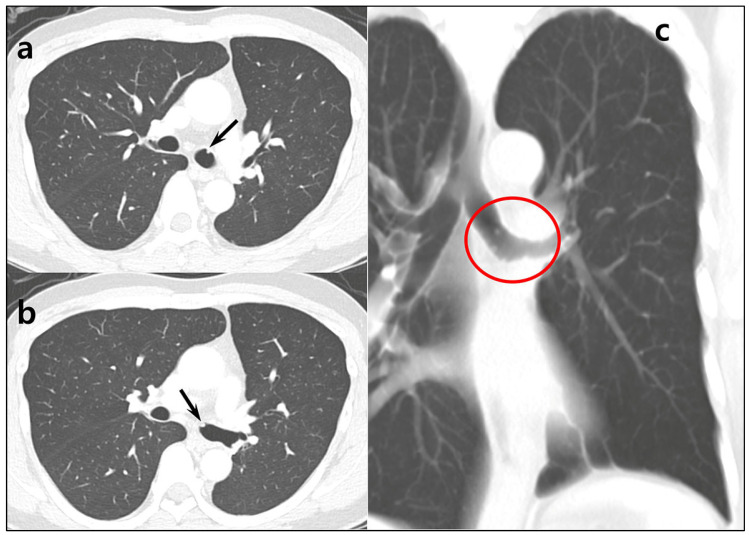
Follow-up CT images obtained in the fifth-year post-operation. (a,b) Chest CT revealed several tiny nodular densities in the left main bronchus (arrows). Although the possibility of bronchial secretions was initially considered, the lesions persisted on a 3-month follow-up CT. (c) A coronal thick slab reformatted image provided a clearer view of multiple nodular lesions, each measuring less than 5 mm (red circle).
